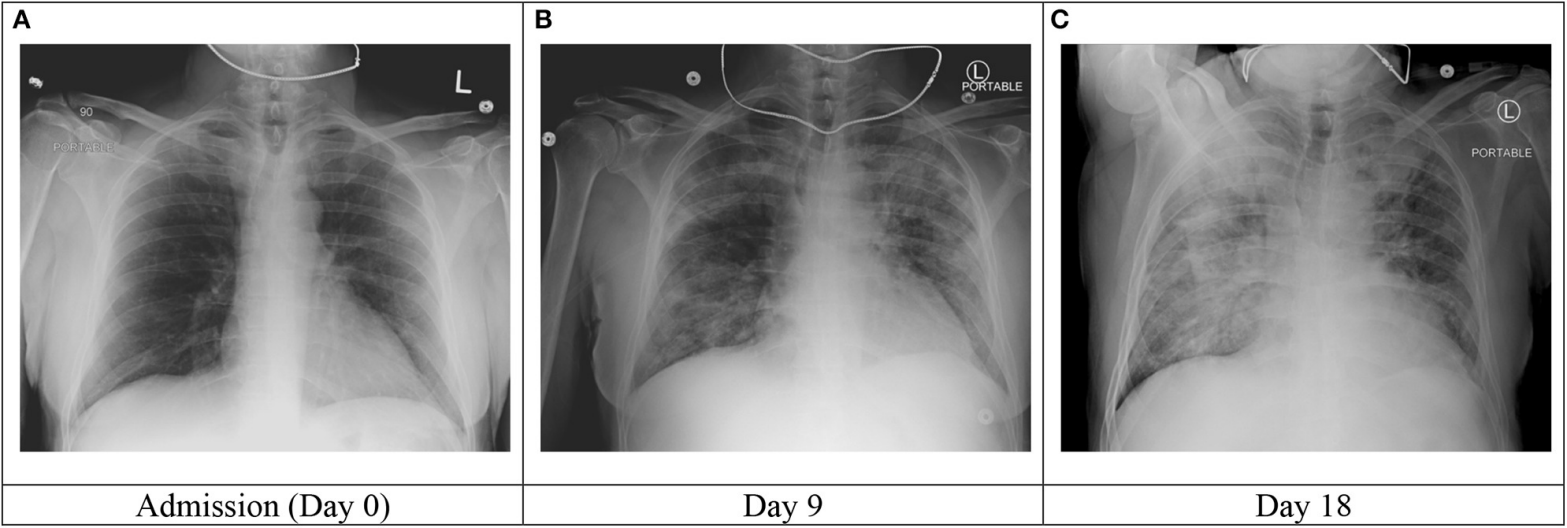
Select chest radiograph images during the patient's hospitalization.
